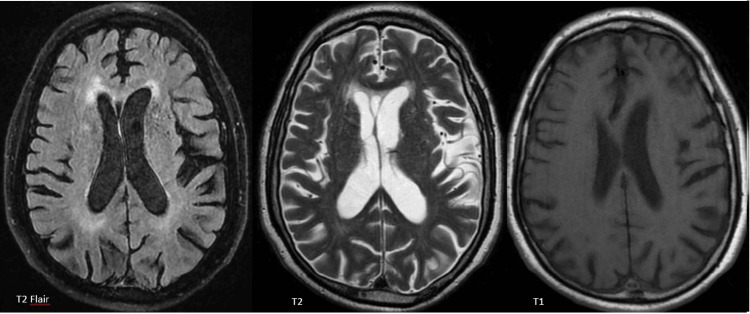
Re-evaluation magnetic resonance images at six months with resolution of previous lesions
